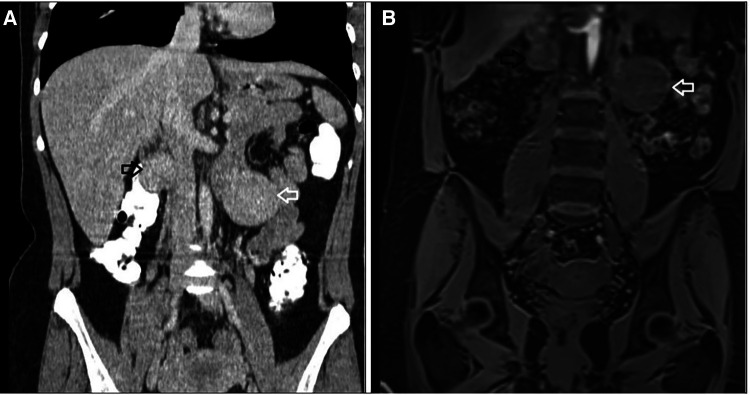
Imaging before the first surgery where both tumors are apparent. (A) Computed Tomography with intravenous contrast, coronal view, (B) MRI, coronal view: white arrow indicating the 4.1 cm retroperitoneal mass located in the left anterior pararenal space, black arrow indicating the tumor located in the right retroperitoneal space.
